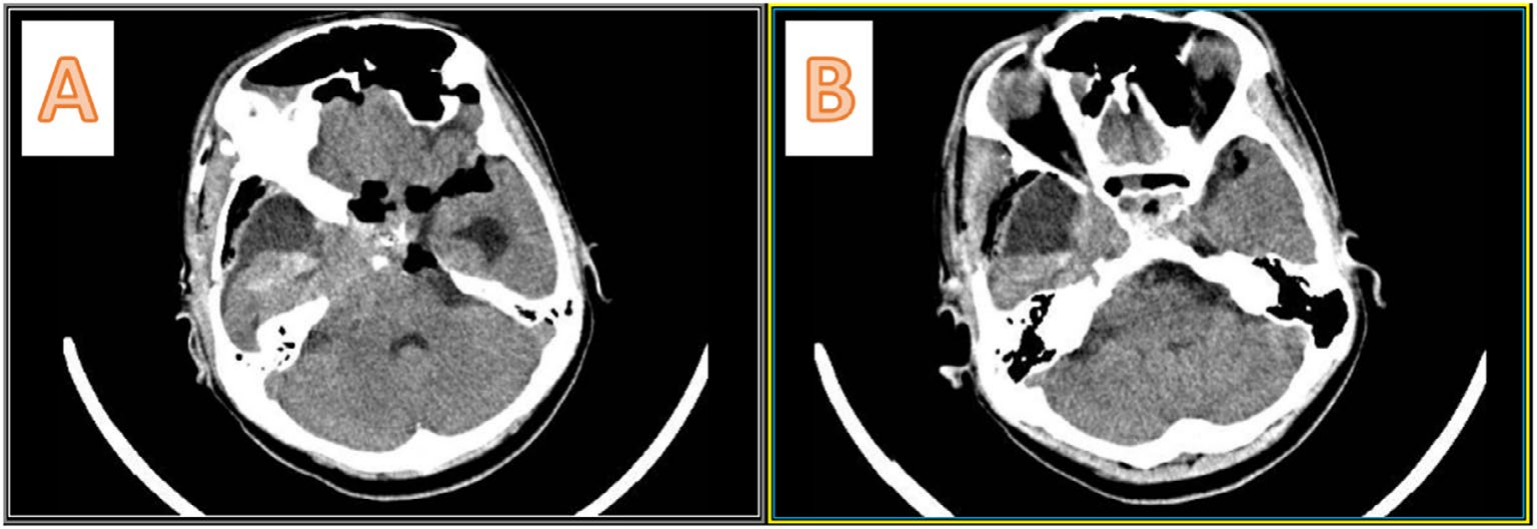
Postoperative non‐contract enhanced brain CT scan. (A, B) Subtotal resection of the mass with complete removal of the middle fossa and sella components and remnant of the posterior fossa component.
